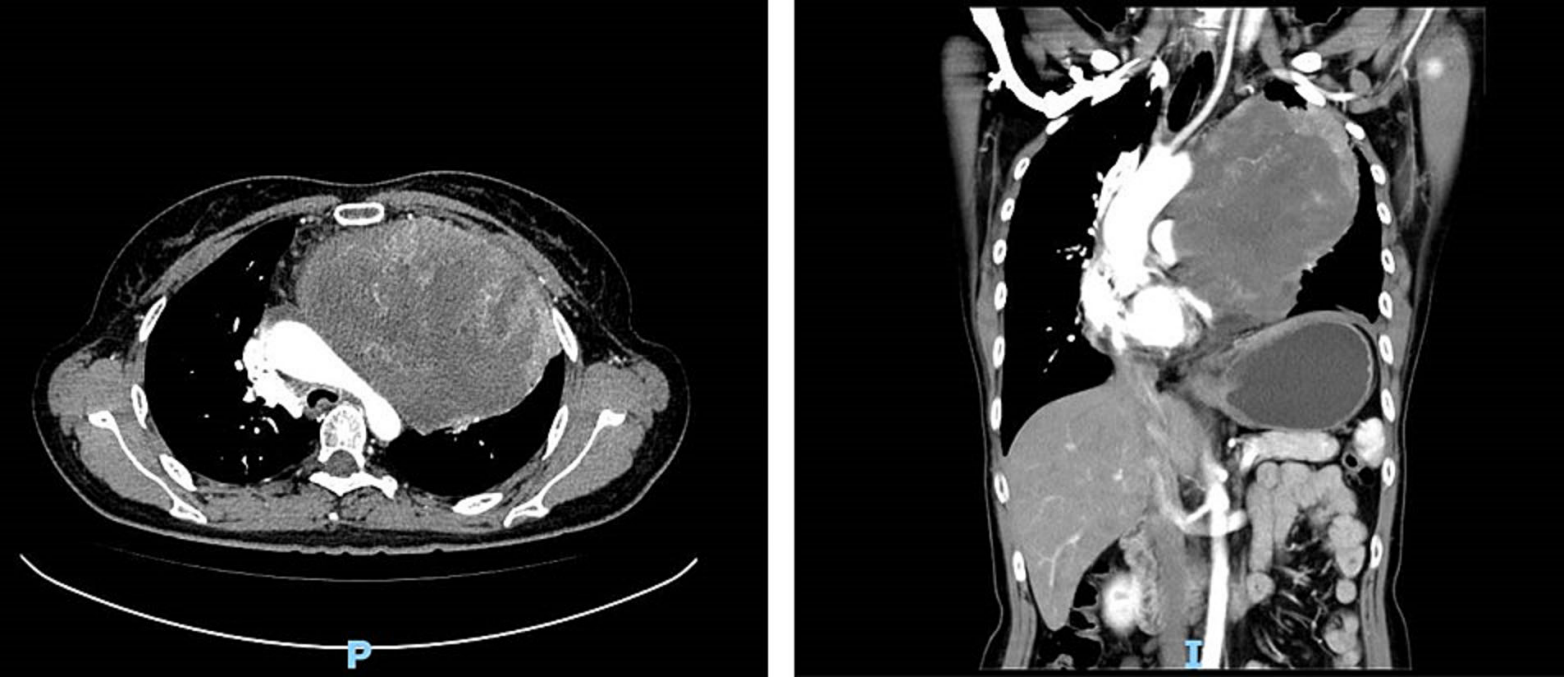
A contrast‐enhanced chest computed tomography scan revealing a large, heterogeneously enhancing, well‐circumscribed, oval mass located adjacent to the left middle and upper mediastinum. The mass, with dimensions of 15 × 12 × 14 cm, comprises both solid and cystic components.
